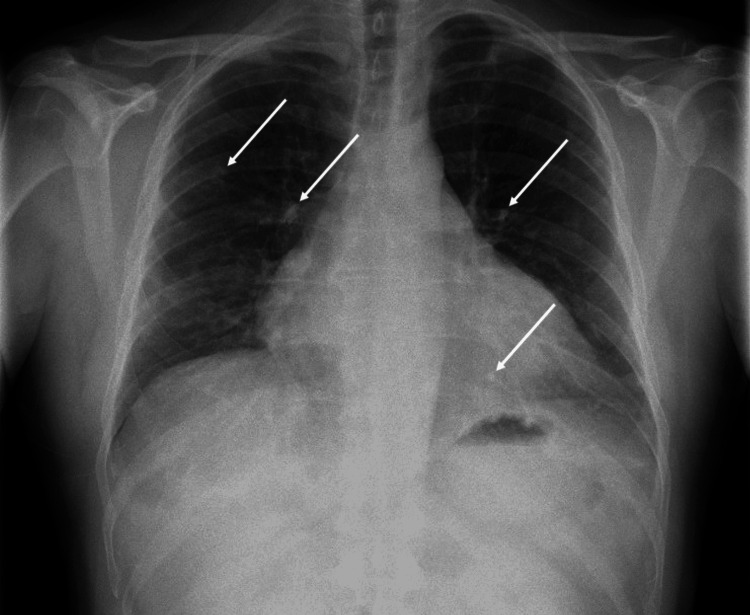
Admission chest X-ray identifying multifocal nodular opacities in both lung fields, stable cardiomegaly, and no pleural effusion
